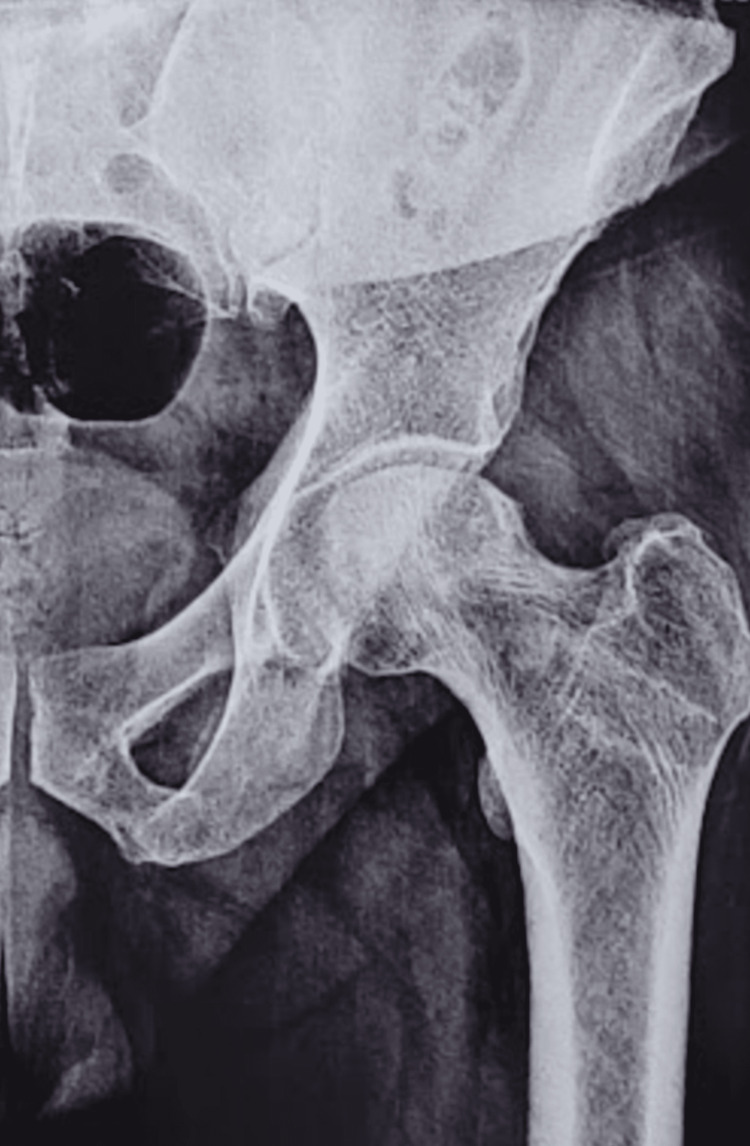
Three-month postoperative anteroposterior view X-ray of the left hip
